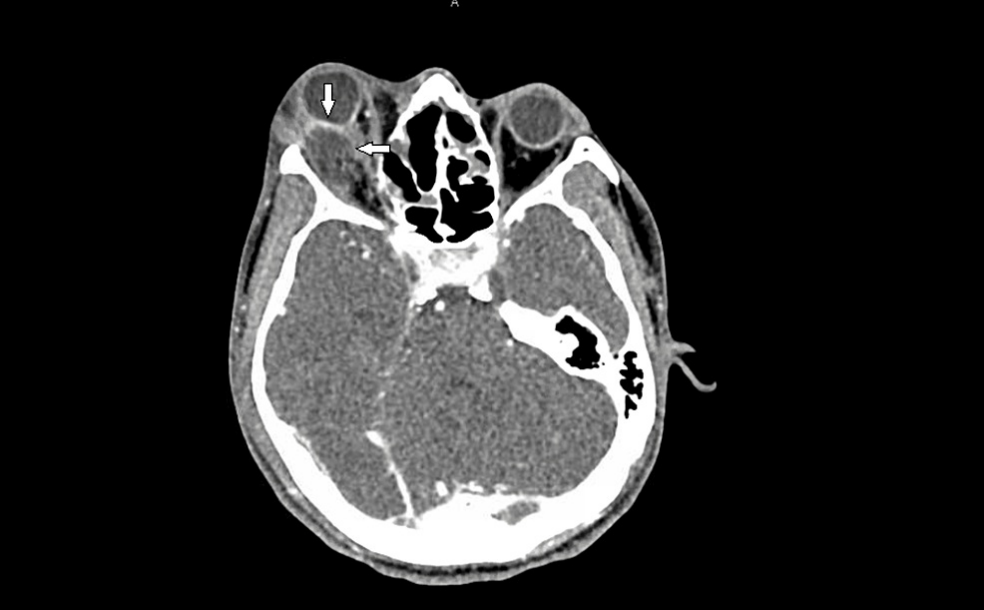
CT Orbit from the second visit showing an axial view with a right convex orbital abscess arising from the lateral wall of the orbit and obscuring the view of the lateral rectus with deformity noted to the globe and compression of the optic nerve.
